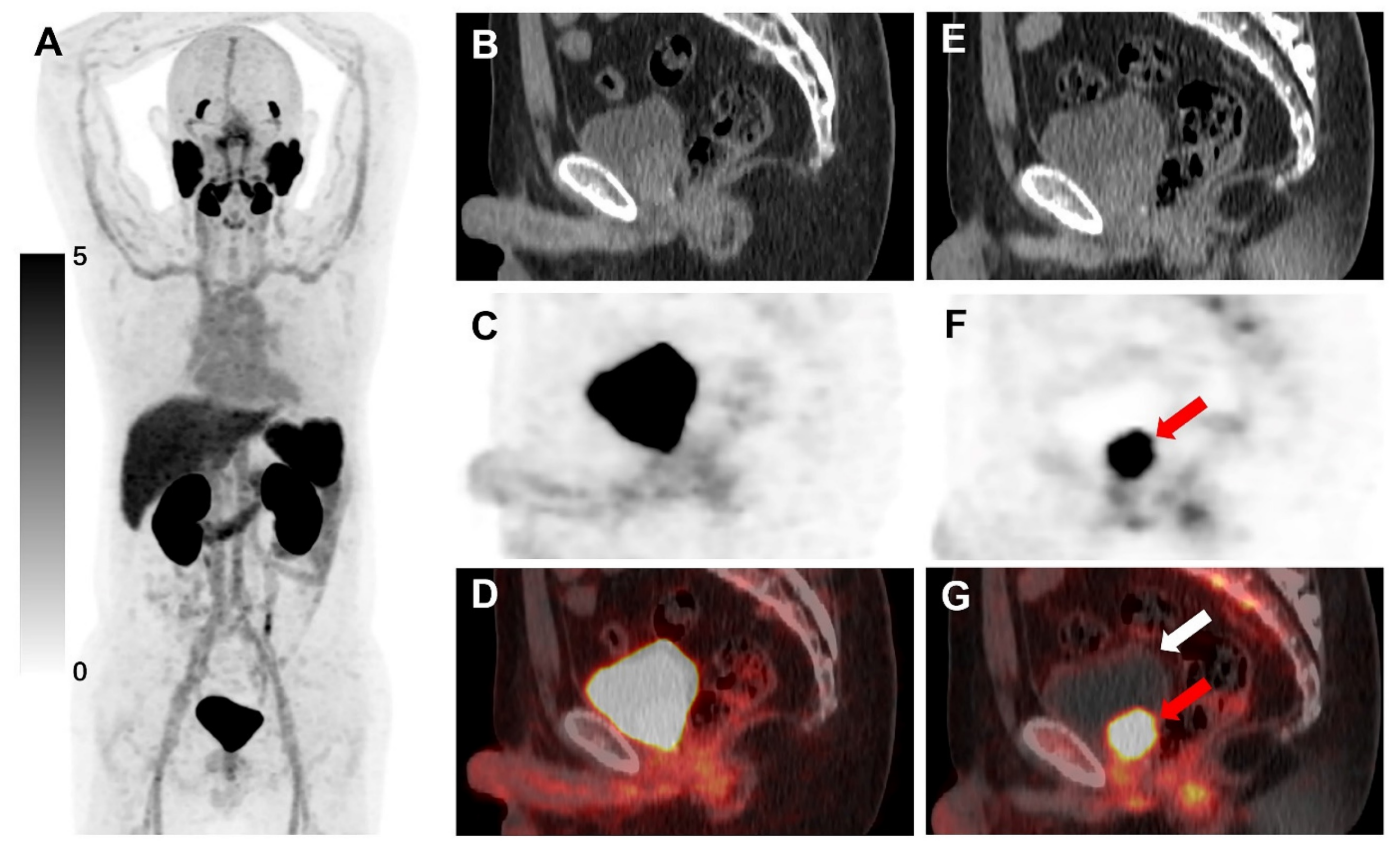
[^18^F]AlF-Thretide PET/CT images were acquired from a 54-year-old man with PCa who experienced biochemical recurrence after radical prostatectomy. His total PSA level was 0.26 ng/mL before the PET/CT scan. (A-D) The routine PET/CT scan conducted 60 min post-injection showed that the SUVmax of the local recurrence (SUVmax 38.1) was higher than that of the bladder (SUVmax 21.7). In our study, this local recurrence was not considered obscured by urinary uptake on the routine scan. However, tracer accumulation in the bladder would have increased the likelihood of missing this lesion in clinical practice. (E-G) The early PET/CT scan, conducted 182 s post-injection, clearly showed this local recurrence with significant tracer uptake (red arrow), compressing the urinary bladder. No tracer accumulation was observed in the urinary bladder at this time (white arrow). This local recurrence showed a significant reduction in volume after radiotherapy.
